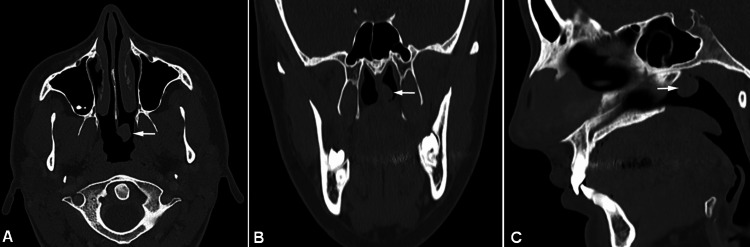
Sinus CT scan A polypoid mass (white arrow) (11x9x15mm) deriving from the nasal septum with no invasion of the surrounding structures. Mild enhancement of the mass after intravenous contrast agent administration. A. Axial, B, Coronal, C. Sagittal
